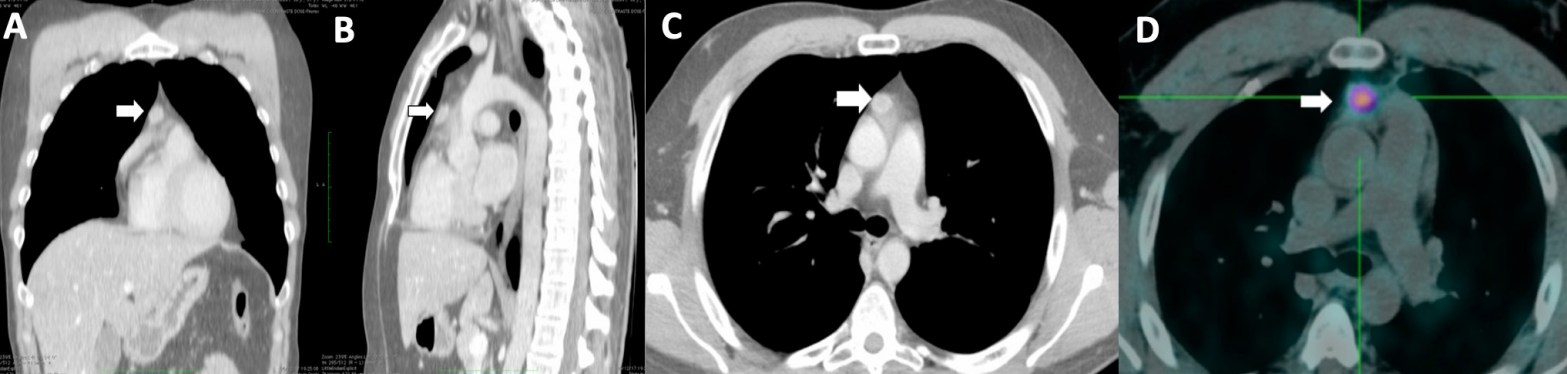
Chest computed tomography scan in the coronal (
